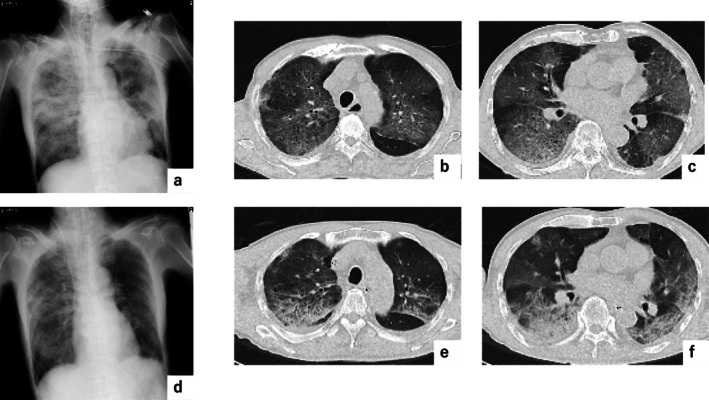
Chest x‐ray and CT findings on admission (a–c) and 2 days after admission (d–f).
